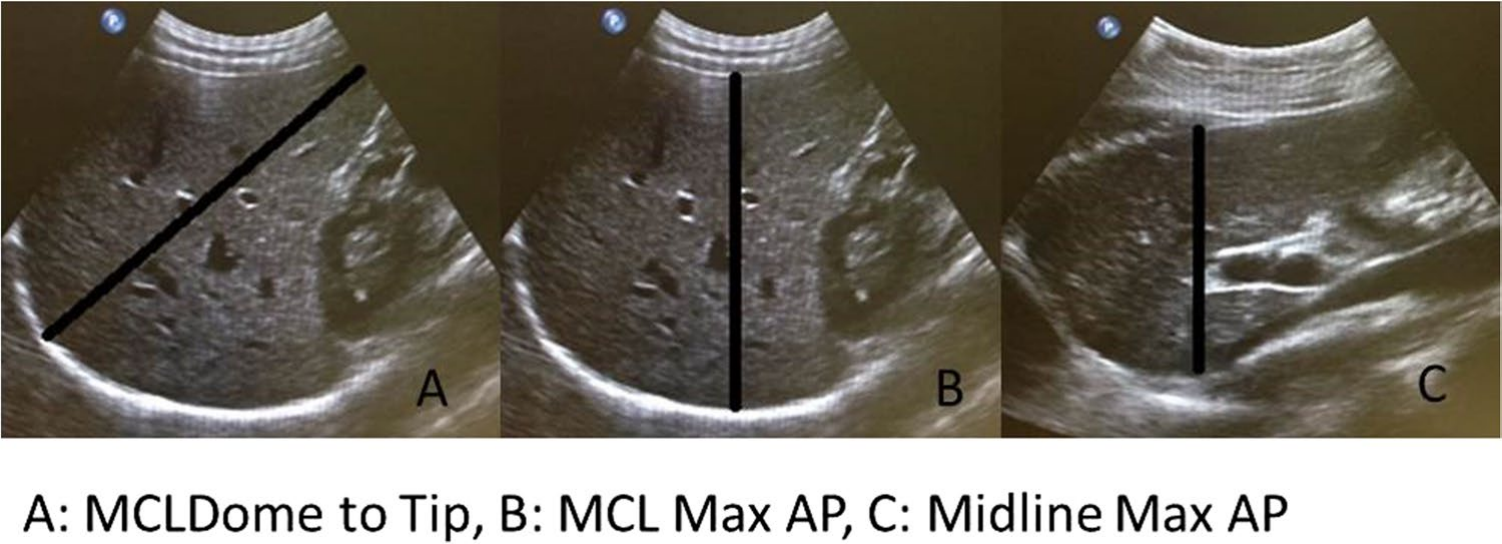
Ultrasound liver measurements where A = mid-clavicular line, dome to the tip of the liver; B = mid-clavicular line, anterior to posterior at liver’s maximum dimension; C = midline, anterior to posterior of the left lobe of liver
